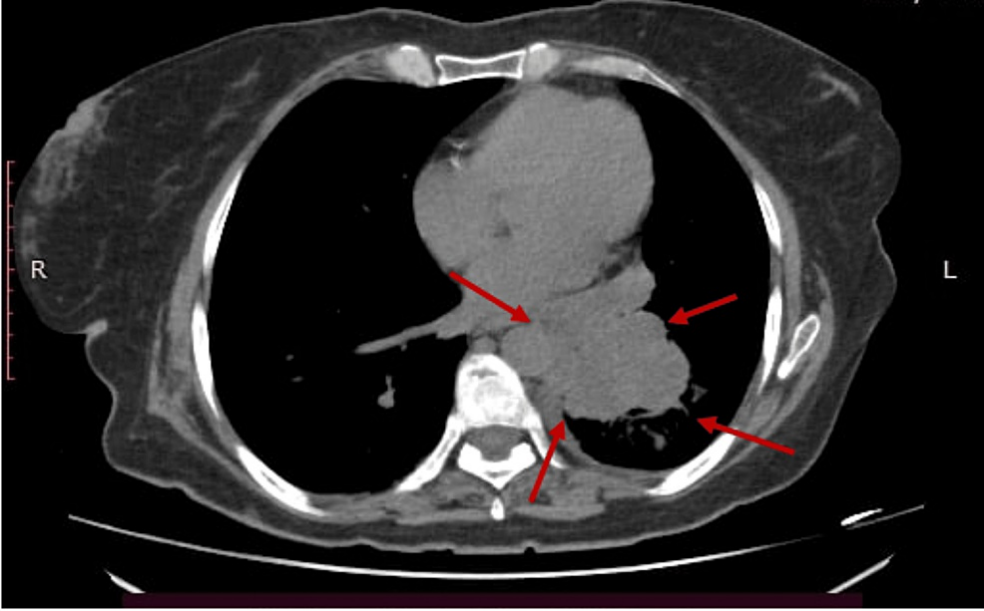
CT scan showing the left lower lobe lung mass
